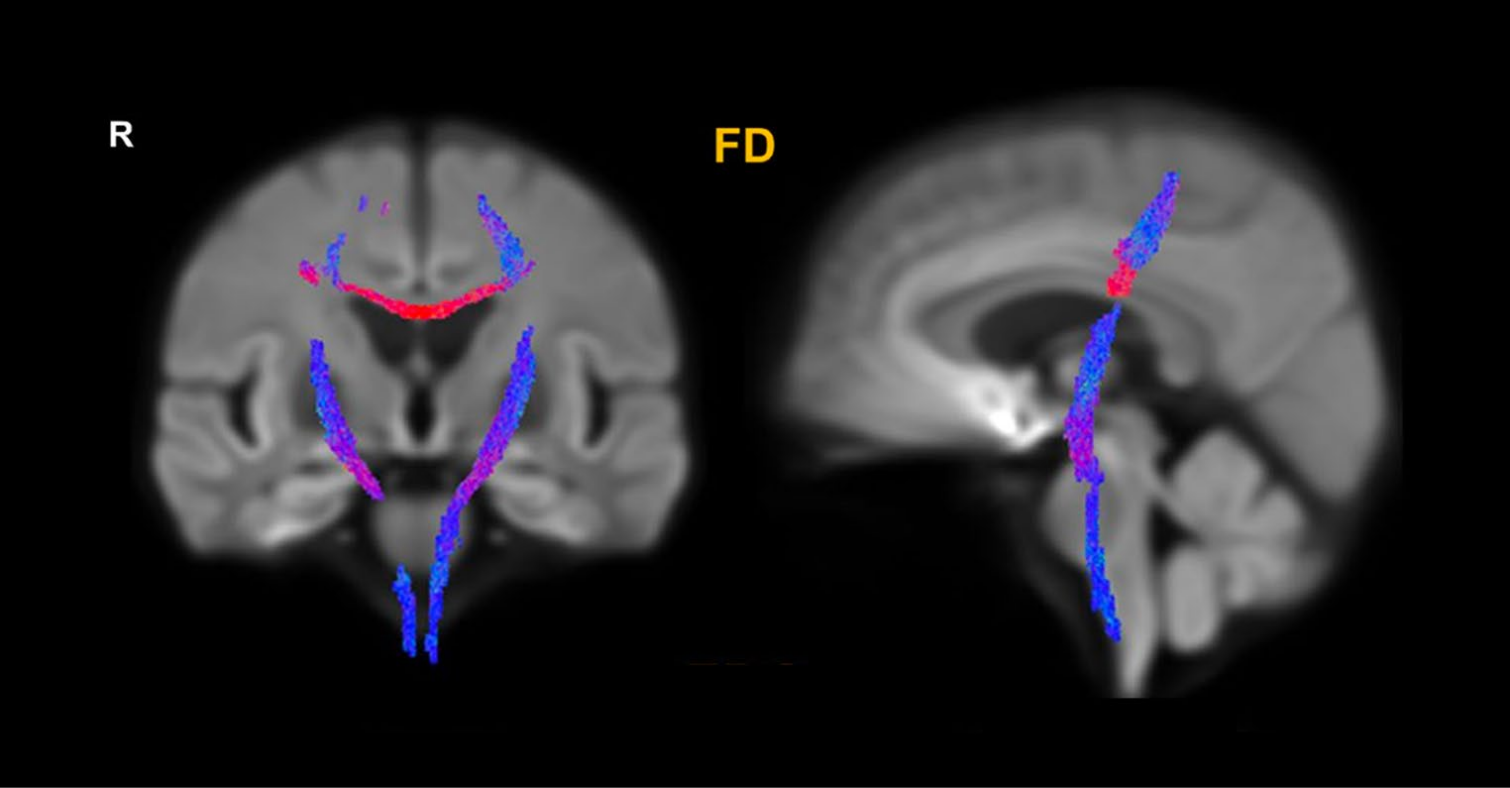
An example of WM macrostructural involvement in ALS. Results of a fixel-based analysis of dMRI data showing a significantly decreased fiber density in the CSTs and the CC in ALS. Reprinted with permissions from [127]. ALS: amyotrophic lateral sclerosis; WM: white matter; dMRI: diffusion magnetic resonance imaging; CST: corticospinal tract; CC: corpus callosum
